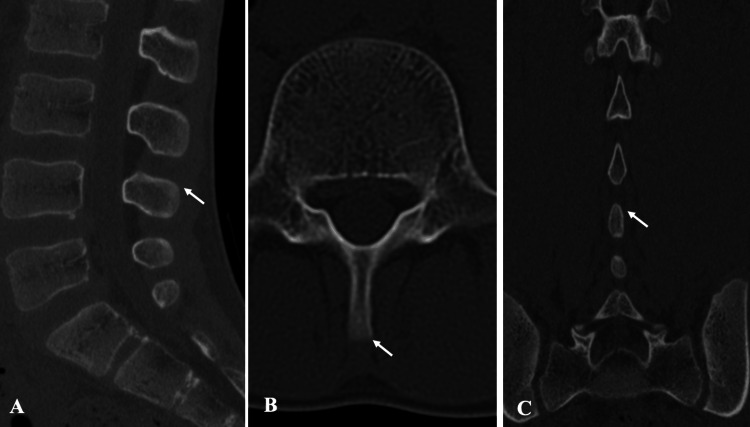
CT findings in Case 2 three months after treatment. CT confirms bony union at the fracture site: (A) sagittal view, (B) axial view, and (C) coronal view. CT, computed tomography
